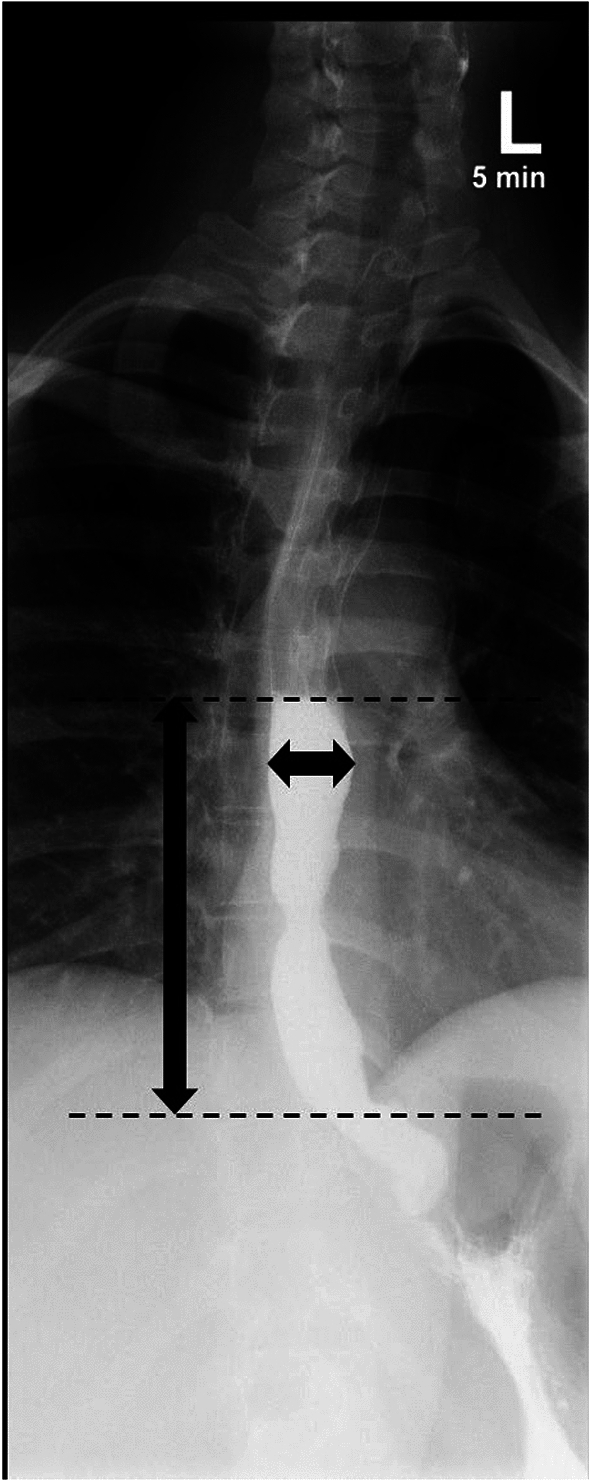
Following ingestion of barium, an LPO radiograph at 5 min demonstrates a columnation of contrast. Vertical arrow shows column height. Horizontal arrow shows column width
